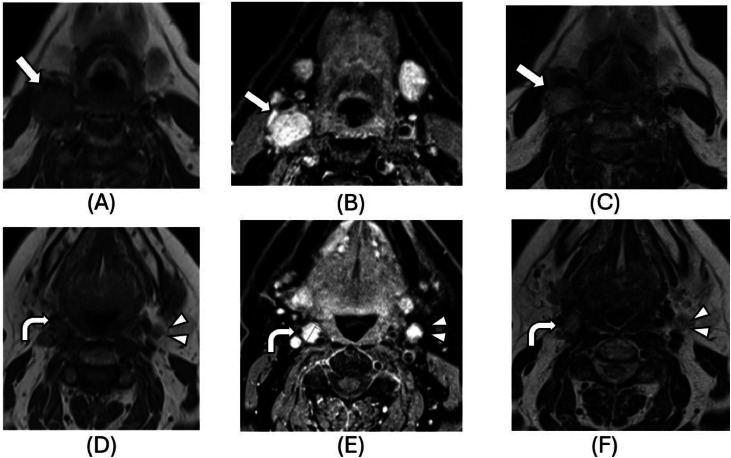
(A-F): Magnetic resonance imaging of the neck in April 2023. Axial T1WI pre-contrast (A, D) and post-contrast (B, E), and axial T2WI (C, F) images below the level of carotid bifurcation (A, B, C, F) and at the level of carotid bifurcation (D, E) demonstrating homogeneously enhancing soft tissue mass (arrows, A-C; curved arrows, D-F), of which demonstrate T2 hyperintensity and contrast enhancement in keeping with classical imaging findings of paraganglioma. Similar rounded nodules in the left level IIA station (arrowheads, D-F) and near the dorsal margin of the right carotid sheath at C1-C2 (not shown) are suspicious for a similar etiology.
